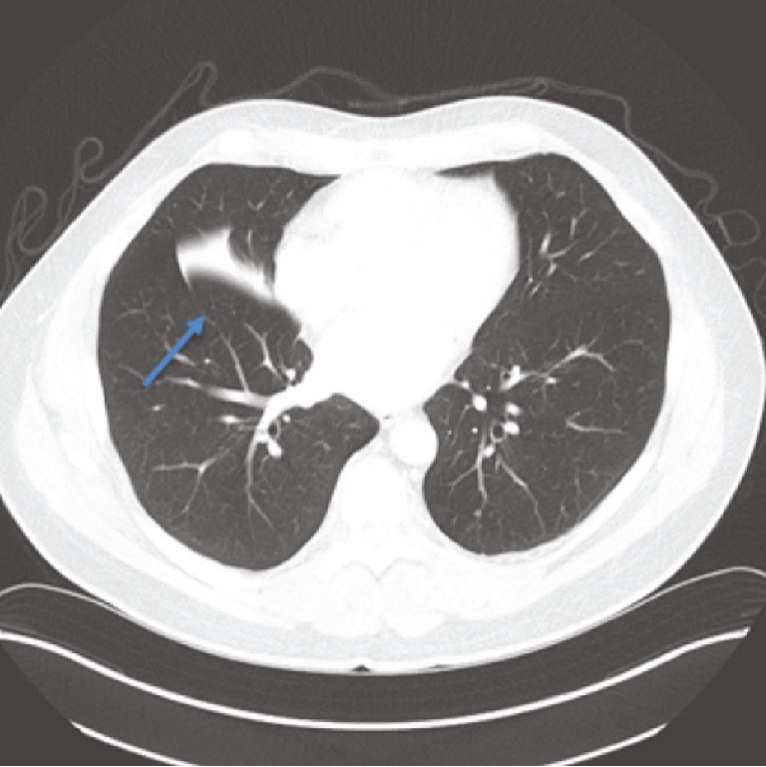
CT chest, axial view: blue arrow denoting an opacity throughout much of the right middle lobe.
